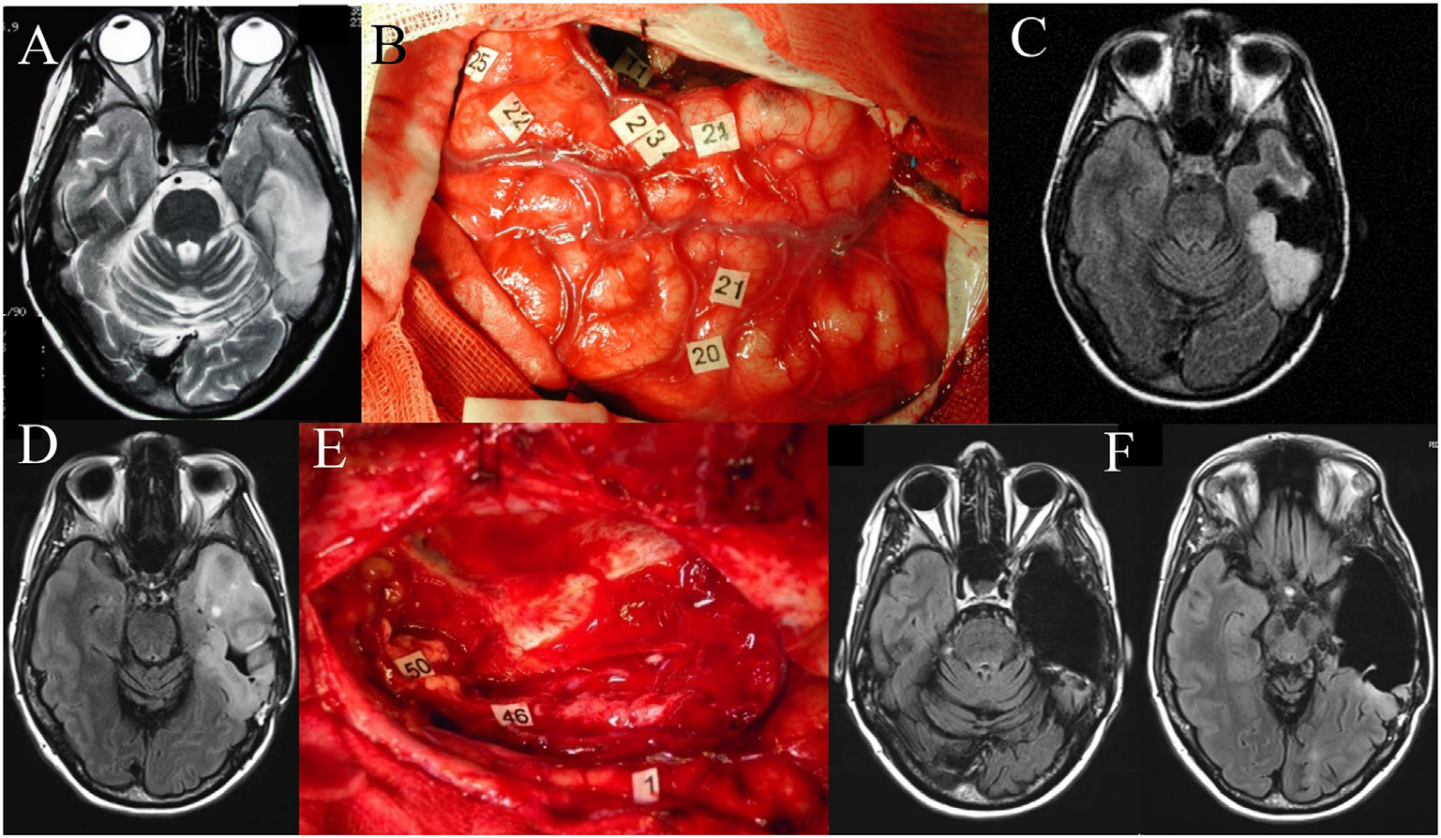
Left temporal diffuse low-grade glioma (DLGG). Axial FLAIR-weighted MRI (A) showing a left temporal DLGG in a 36-year-old patient who presented with isolated seizures and no neurological deficits. Intraoperative photograph during the first awake surgery (B), after resection was performed according to individual functional boundaries. Stimulation mapping demonstrated the persistence of eloquent cortical areas in the temporal lobe (tags 22, 23, 24, 25) as well as subcortical fibers (tag 11, corresponding to the inferior longitudinal fascicle) still critical for language function. Postoperative axial FLAIR-weighted MRI (C) revealing a partial resection, with a posterior residual tumor voluntarily left for functional reasons. The diagnosis of DLGG was confirmed, and the patient resumed a normal familial, social, and professional life. Ten years later, epileptic seizures recurred concomitantly with an imaging progression as demonstrated on the axial FLAIR-weighted MRI (D). Reoperation was proposed to the patient. Intraoperative photograph (E) during the second awake surgery, after resection was performed according to the new individual functional boundaries. Electrocortical stimulation mapping revealed brain reorganization, allowing the achievement of a significantly wider resection compared to the first surgery. Of note, at the subcortical level, stimulation of the left inferior fronto-occipital fascicle (IFOF) (46 and 50) elicited semantic paraphasia when stimulated at the end of surgery. Thus, resection of the anterior part of the inferior longitudinal fascicle was possible given the compensation provided by the direct ventral pathway represented by the left IFOF. Postoperative axial FLAIR-weighted MRI (F) performed 3 months after the second surgery showing a complete resection, made possible due to mechanisms of neuroplasticity, in a patient who returned to a normal life with no permanent neurological deficit.
